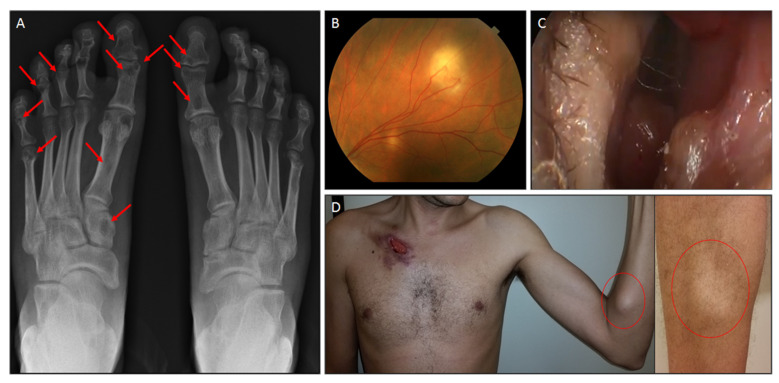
Sporotrichosis multifocal involvement. (A) Radiography of the feet of a 36-year-old man, with AIDS and low compliance to treatment. Several rounded to oval osteolytic lesions (red arrows point some) are seen on both feet. (B) Fundus image showing yellowish choroidal lesions. (C) Right nasal fossa-Infiltration in the right inferior turbinate head, nasal vestibule and superior septal region. (D) A 41-year-old previously healthy man with the cutaneous disseminated form of sporotrichosis. Ulcerated lesion surrounded by purplish lesions in the region of the right clavicle. Diffuse painless nodules/masses, here seen in the left forearm and in the right leg (red circles).
